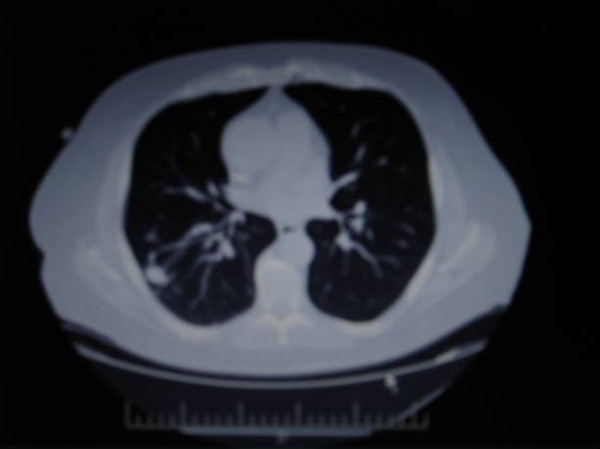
Chest CT scan. Nodule involving the right lower lobe, laterally, measures 1.1 × .6 cm.
